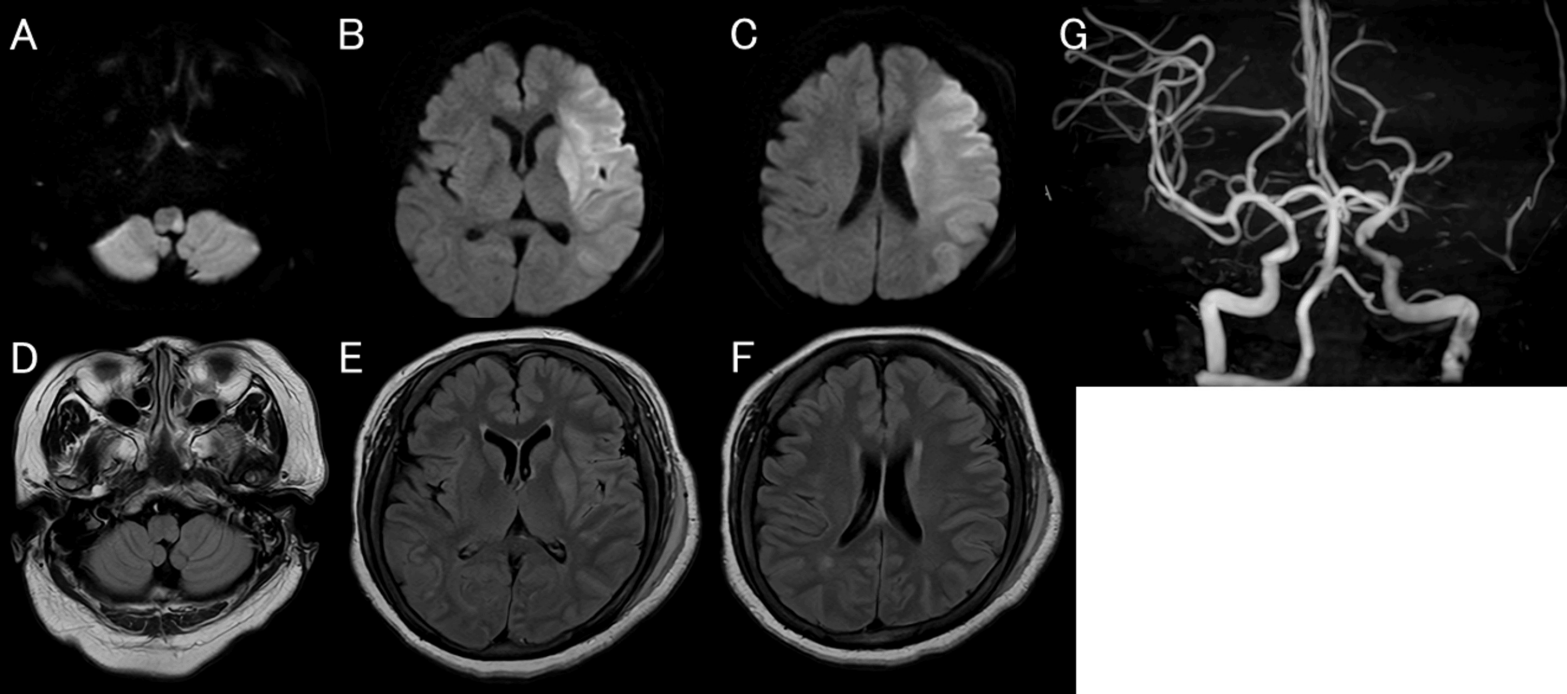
MRI and MRA findings on arrival at our hospital. Diffusion-weighted imaging (DWI) demonstrating acute infarction in the left lateral medulla and left middle cerebral artery territory (A-C). Fluid-attenuated inversion recovery (FLAIR) showing no corresponding signal change (D-F), indicating a DWI-FLAIR mismatch. Magnetic resonance angiography demonstrating occlusion of the left vertebral artery and left middle cerebral artery, with a signal defect in the left internal carotid artery (G).
